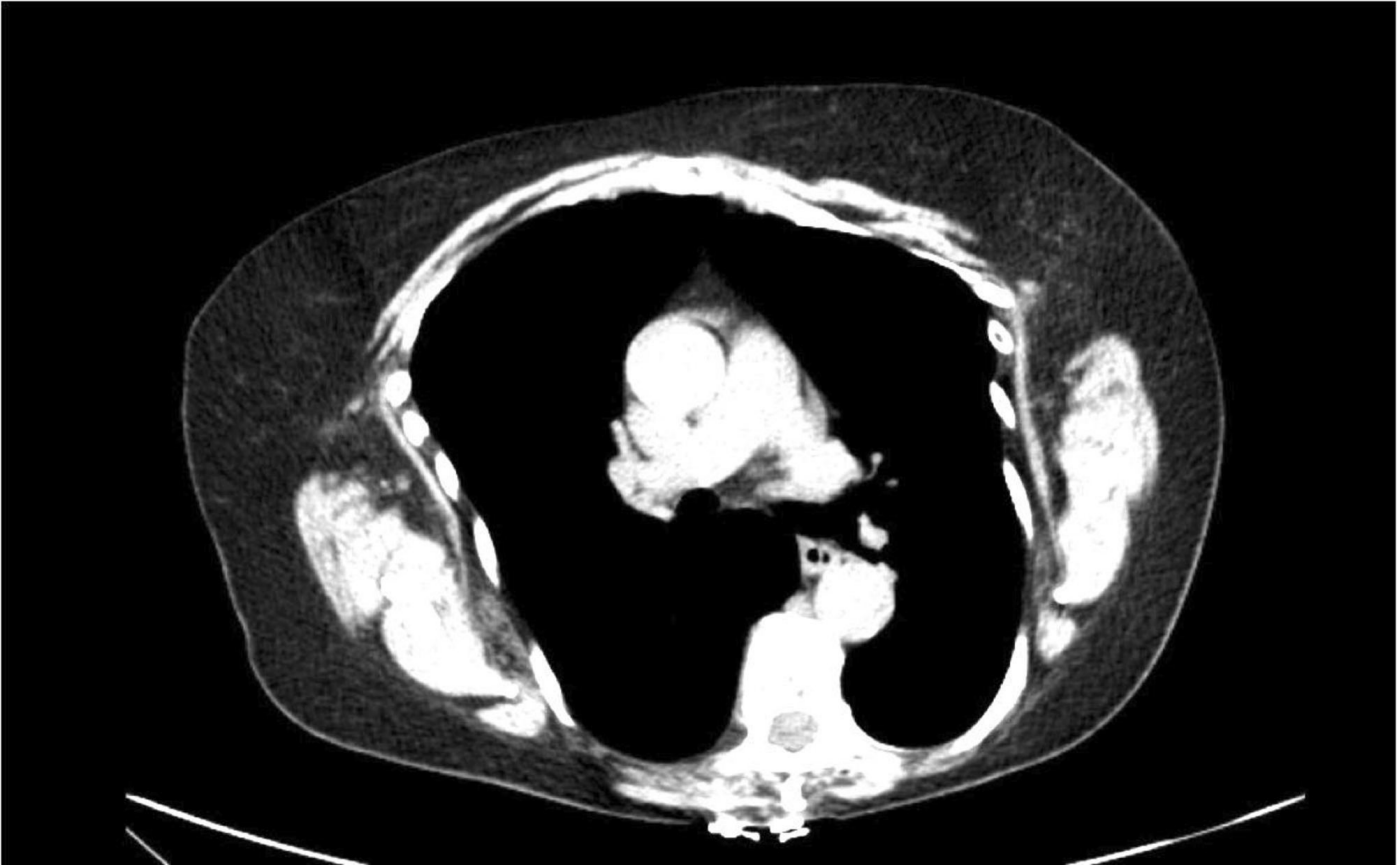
Two-week follow-up chest CT demonstrating resolution of lymphadenopathy CT: computed tomography
